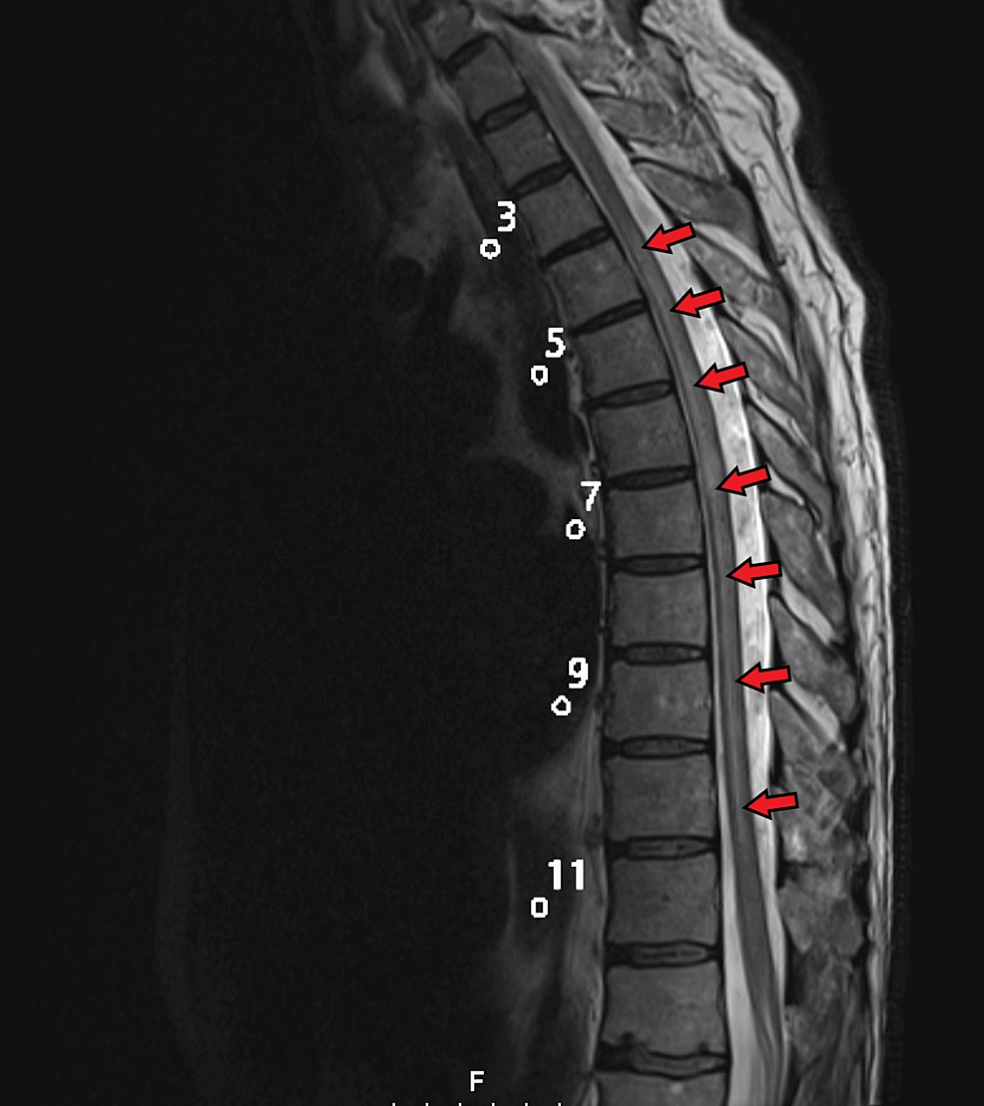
MRI of the thoracic spine (Patient #2). A long segment of high signal in the spinal cord from T3-T4 through T10-T11 without associated enhancement; findings are concerning for transverse myelitis.
